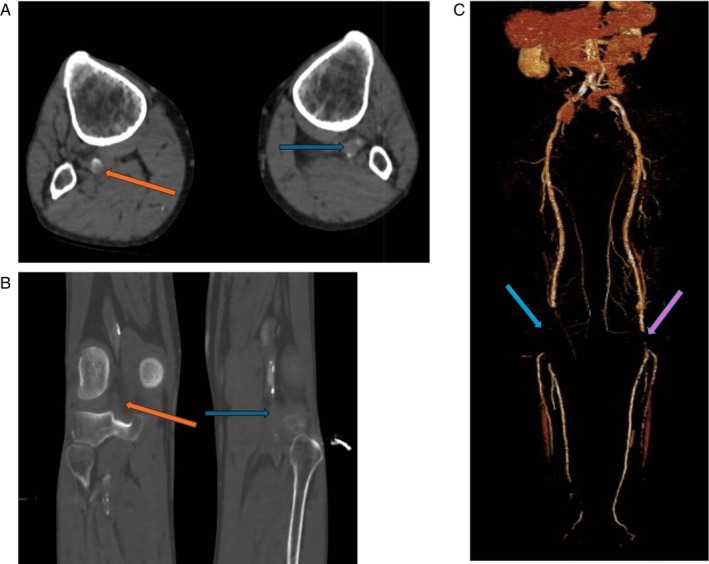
(A) CT‐A with lower limb runoff (further distal) showing occlusion of the right (orange arrow) as well as the left (blue arrow) popliteal artery. This is evident in the lack of contrast in the popliteal arteries shows that there is an occlusion at the level of the popliteal arteries bilaterally. (B) Saggital Views of Popliteal Artery Occlusions: Similarly to the axial views, there is a lack of contrast in the popliteal arteries in this view. (C) 3D Reconstruction of CT‐A of Lower Limbs prior to the patient's second operation. Please note both arrows highlighting the absence of continuity in this 3D reconstruction. On the patient's Right (blue arrow), this is from the origin of the popliteal artery to the origins of the anterior tibial artery and the tibioperoneal trunk. On the left (purple arrow) the second part of the popliteal artery is missing. The lack of continuity in the lower limb vasculature shows the absence of blood flow in the affected areas signifying occlusion.
